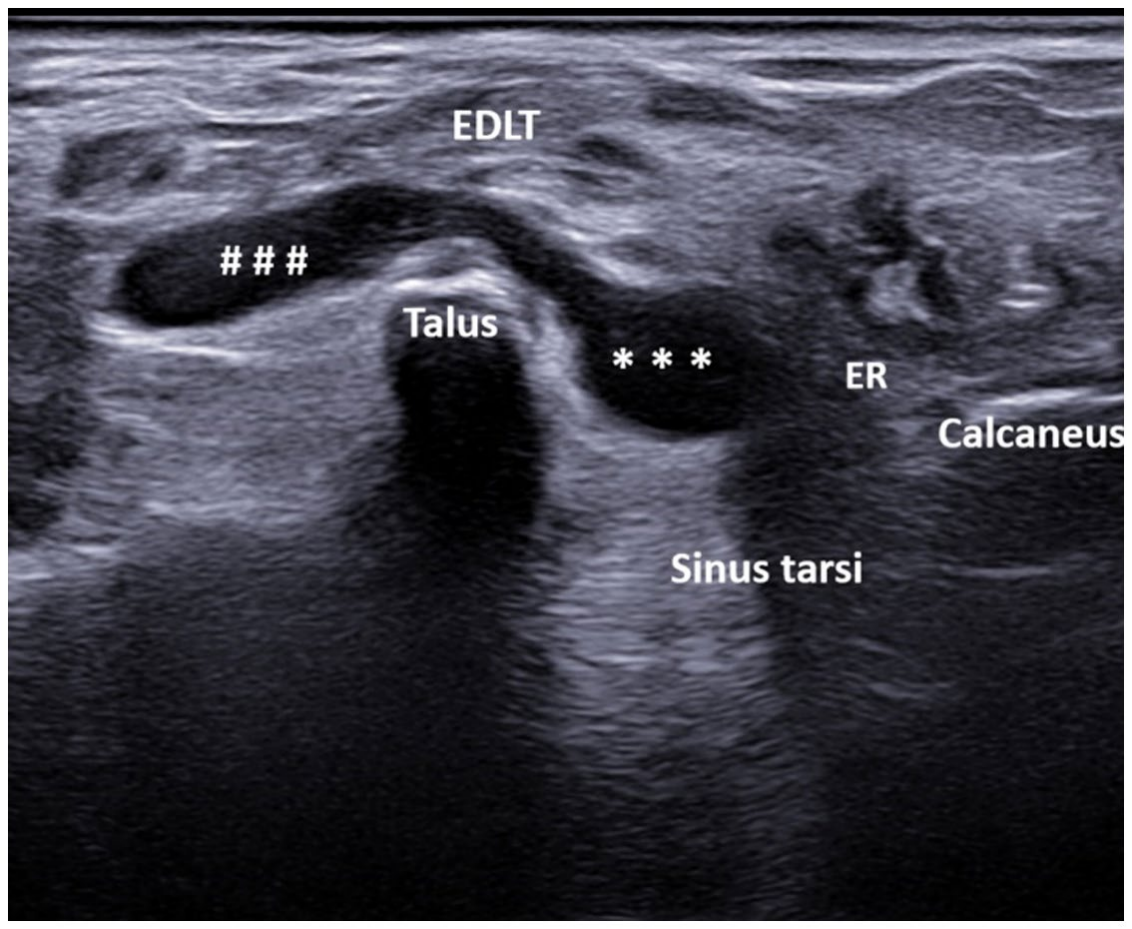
A 44-year-old patient with spondyloarthritis. Ultrasound image showing bilocular GB with the transducer positioned in the short axis of the foot (left side is medial). The transverse ultrasound image of the dorsolateral ankle reveals a bi-lobed, anechoic fluid collection (* – lateral lobe, # – medial lobe) between the extensor digitorum longus tendon (EDLT) and the talus. ER, extensor retinaculum and EDLT, extensor digitorum longus tendon.
